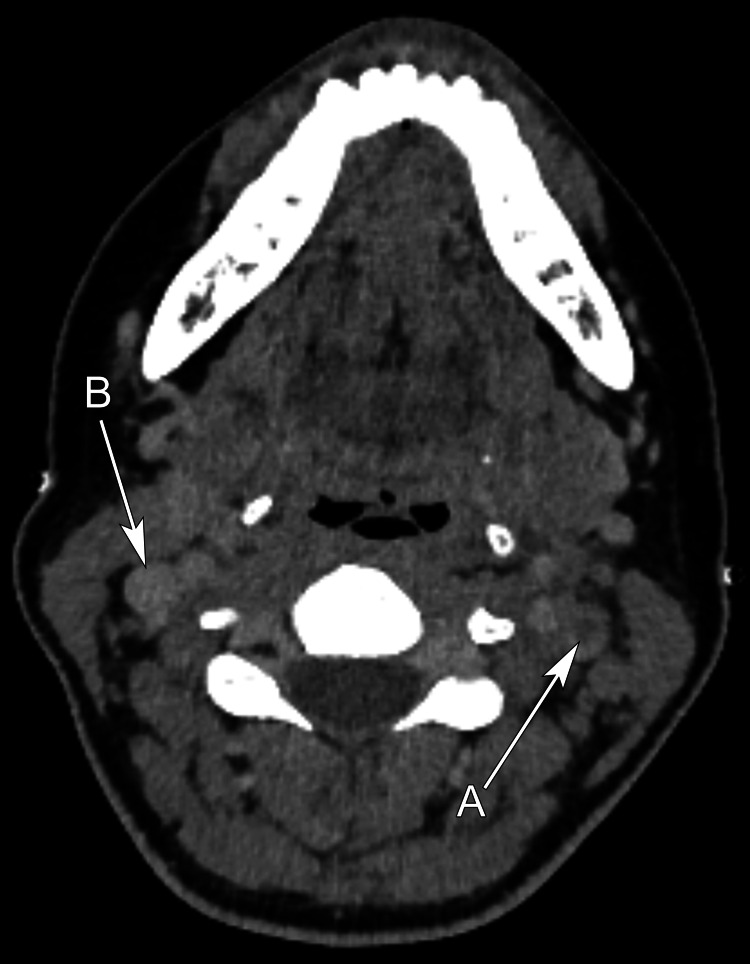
Axial CT postcontrast at the level of the upper neck This confirms a rounded focus of low attenuation in the left internal jugular vein (arrow A) in keeping with thrombus. Note normal enhancing right internal jugular vein (B).
